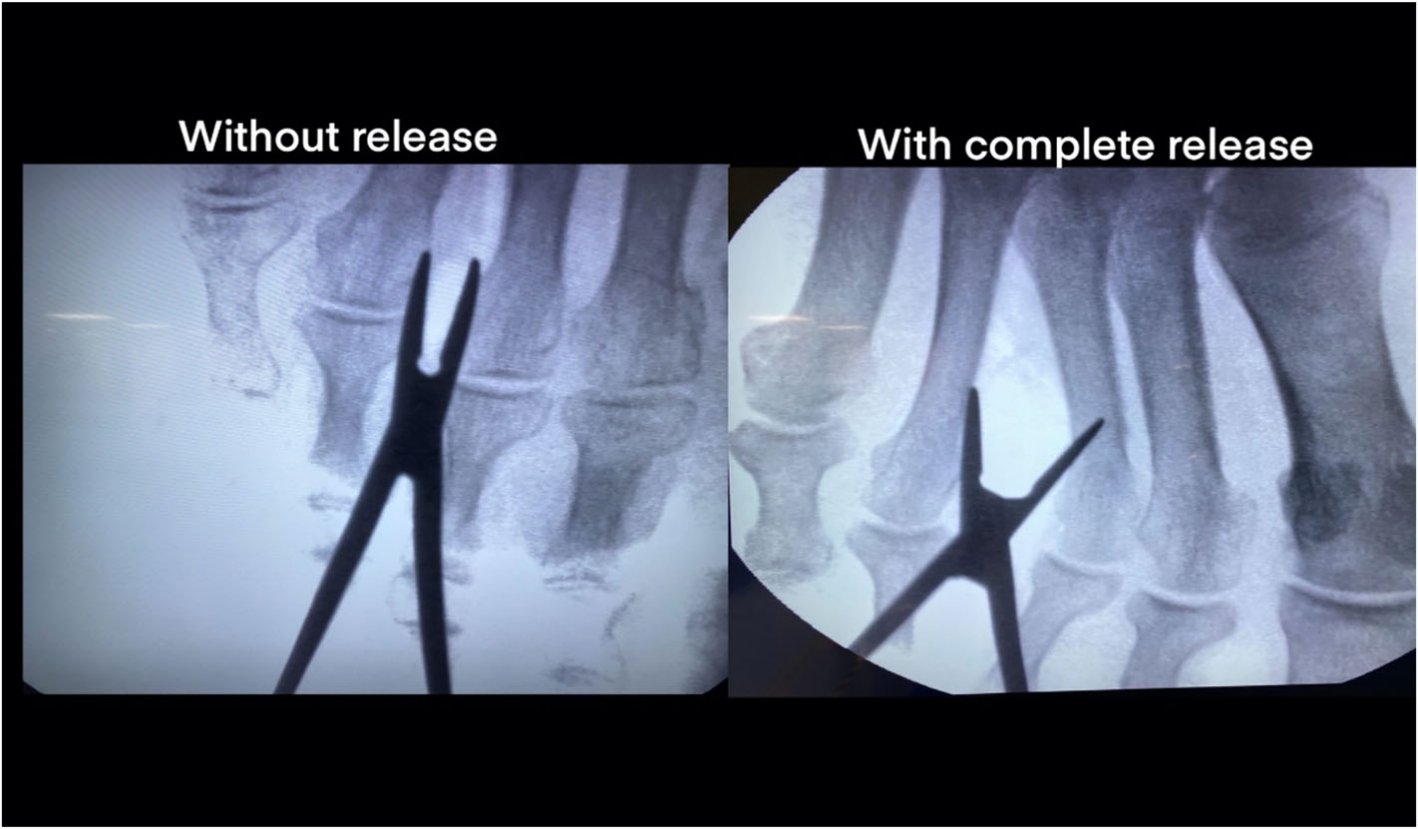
Confirmation of a complete release of the deep transverse metatarsal ligament (DTML) using fluoroscopy—left before, right after the ultrasound‐guided surgical release with widening the third intermetatarsal space [Color figure can be viewed at wileyonlinelibrary.com]
